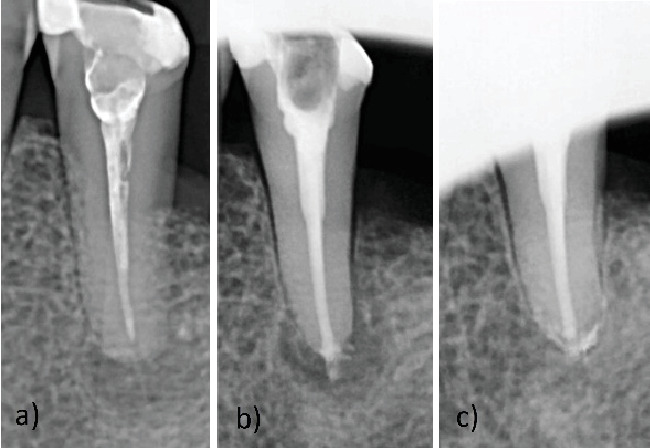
Clinical features of Case Report 3. (a) Preoperative intraoral radiograph. (b) Postoperative intraoral radiograph, moderate extrusion of CS cement. (c) Nineteen-month follow-up, healed periapical lesion with reorganization of the extruded material.
